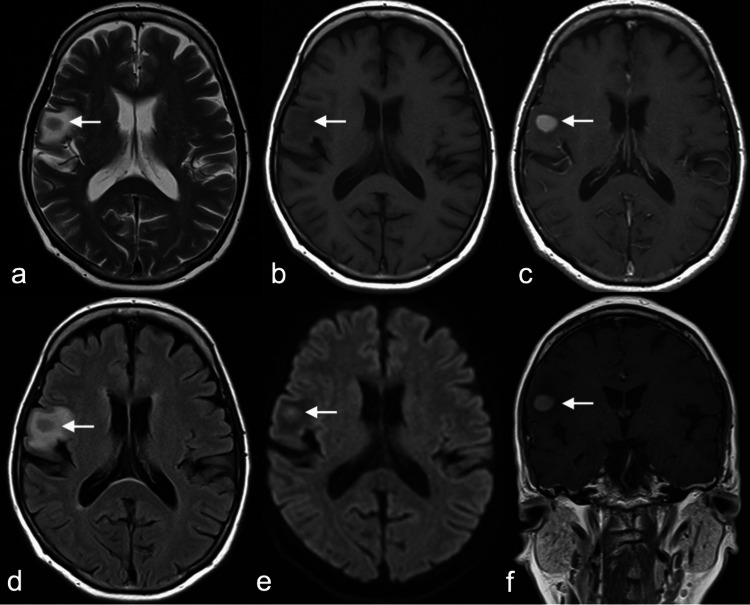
Brain MRI showing the circumscribed lesion involving the right frontal lobe (white arrow) with decreased T2 and T1 signals (a and b). (d) Fluid attenuation inversion recovery image exhibiting mild perilesional vasogenic edema. (e) Diffusion-weighted imaging demonstrating mild restricted diffusion. (c and f) Post-contrast study showing homogenous enhancement of the lesion.
